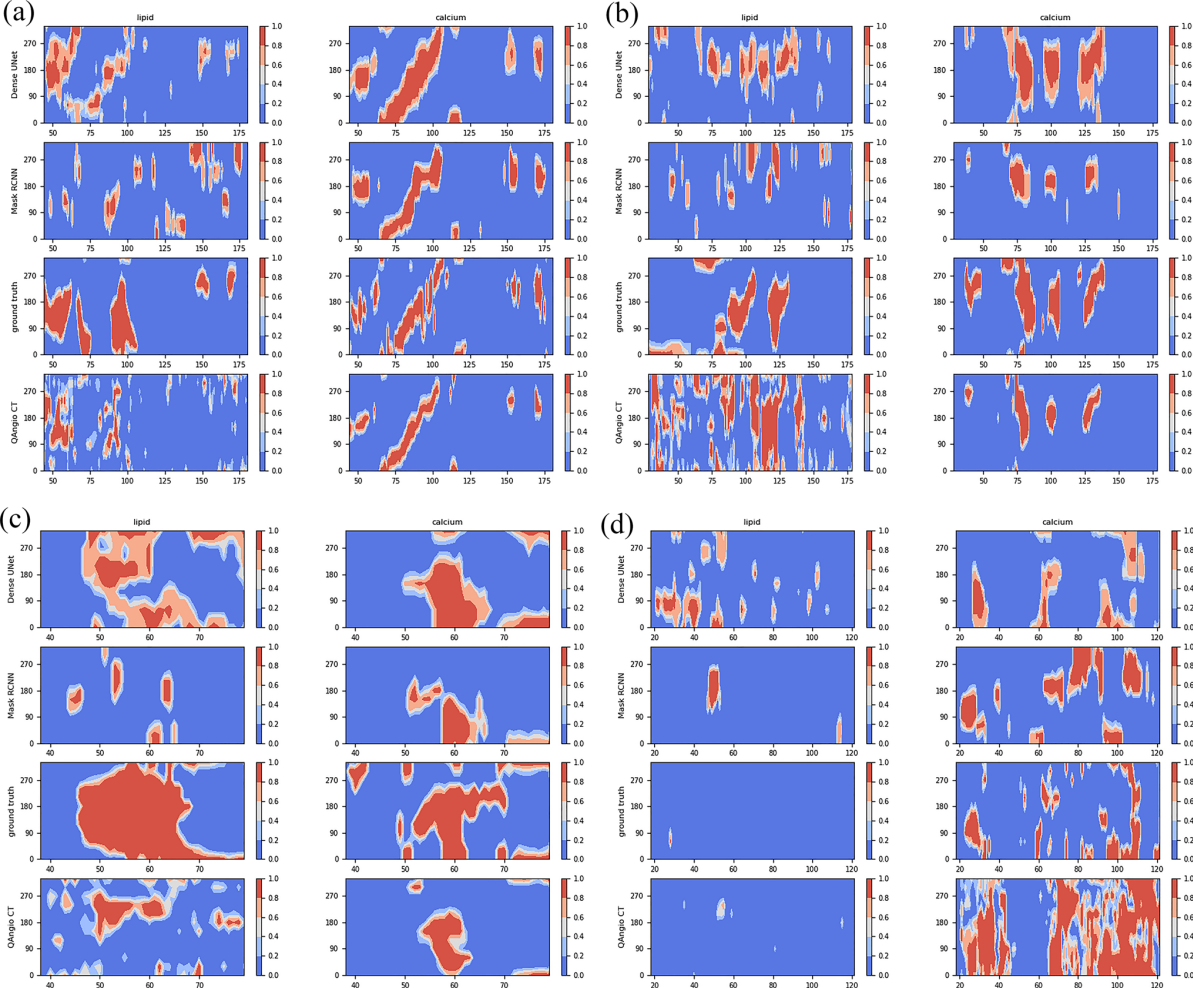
Spread-out view of lipid-rich and calcified plaques of four arteries in the test dataset. The spread-out views were predicted by Dense U-Net, Mask R-CNN, NIRS-IVUS and QAngioCT, respectively. *X*-axis: indices of CT image. *Y*-axis: plaque occurring angle from 0 degree and 359 degree. Color bar: probability of plaque appearance from 0 to 1
